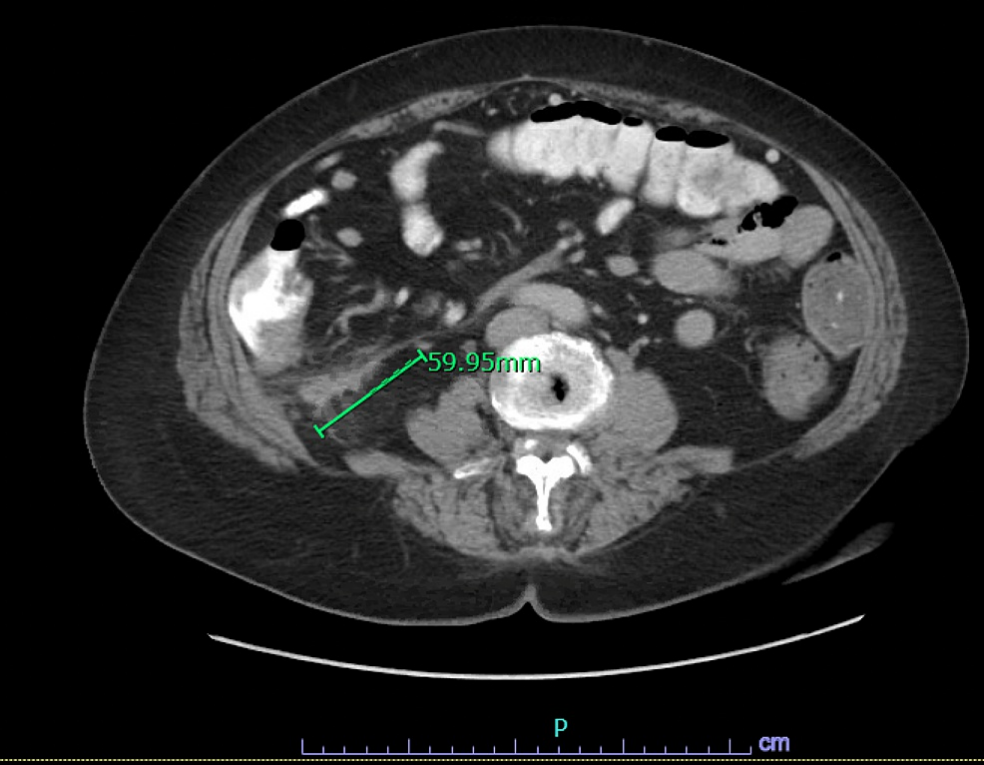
Repeat CT chest abdomen and pelvis show grossly stable 5.9 x 1.7 cm nodular abnormal soft tissue density within the right upper quadrant retroperitoneum posterior to the hepatic flexure.
